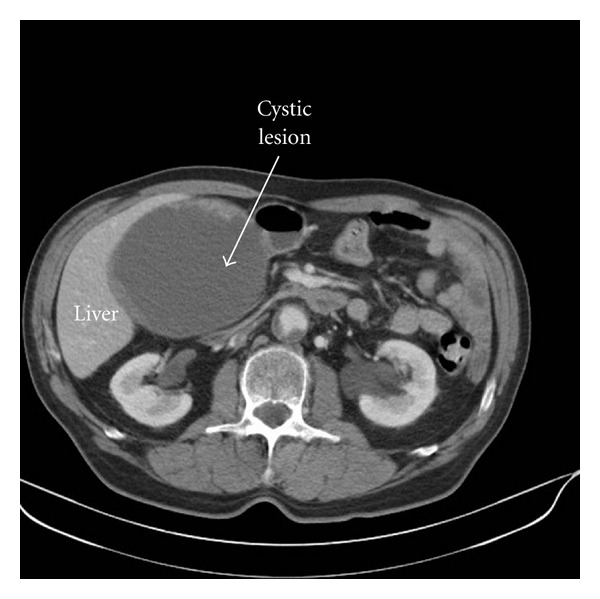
Abdominal computed tomography (CT) displaying a large cystic lesion (arrow) adjacent to the right liver lobe, the stomach, and the pancreatic head.
